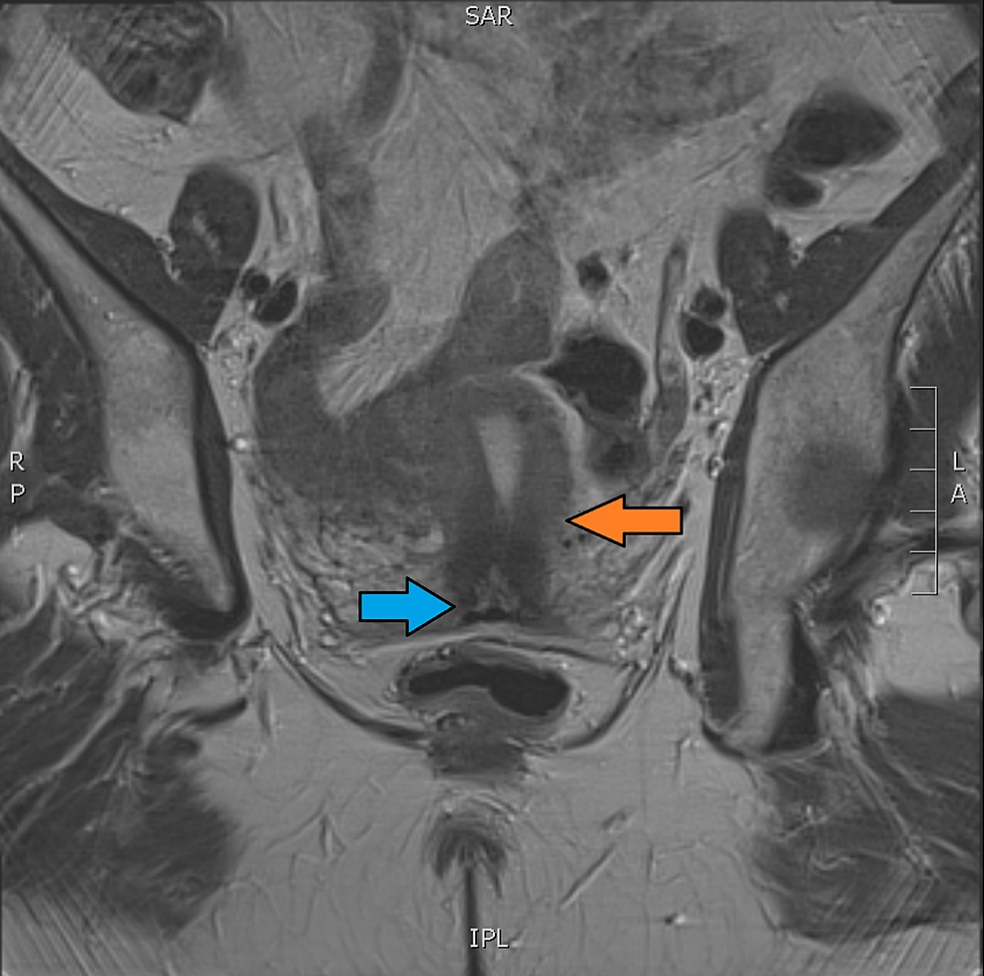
Coronal view from MRI scan Blue arrow: Cervix; Orange arrow: Isthmus of the uterus
